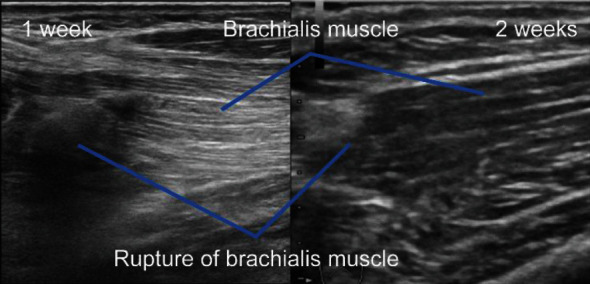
Ultrasound examinations (left: one week after the injury; right: two weeks after the injury).
